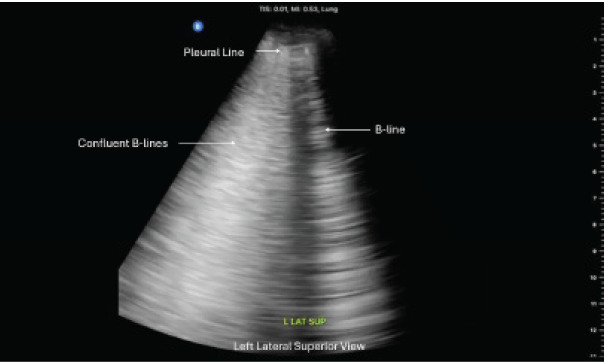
Point-of-care ultrasound of the left lung, in the left lateral superior view, showing confluent and individual B-lines in a non-fatal drowning patient.
